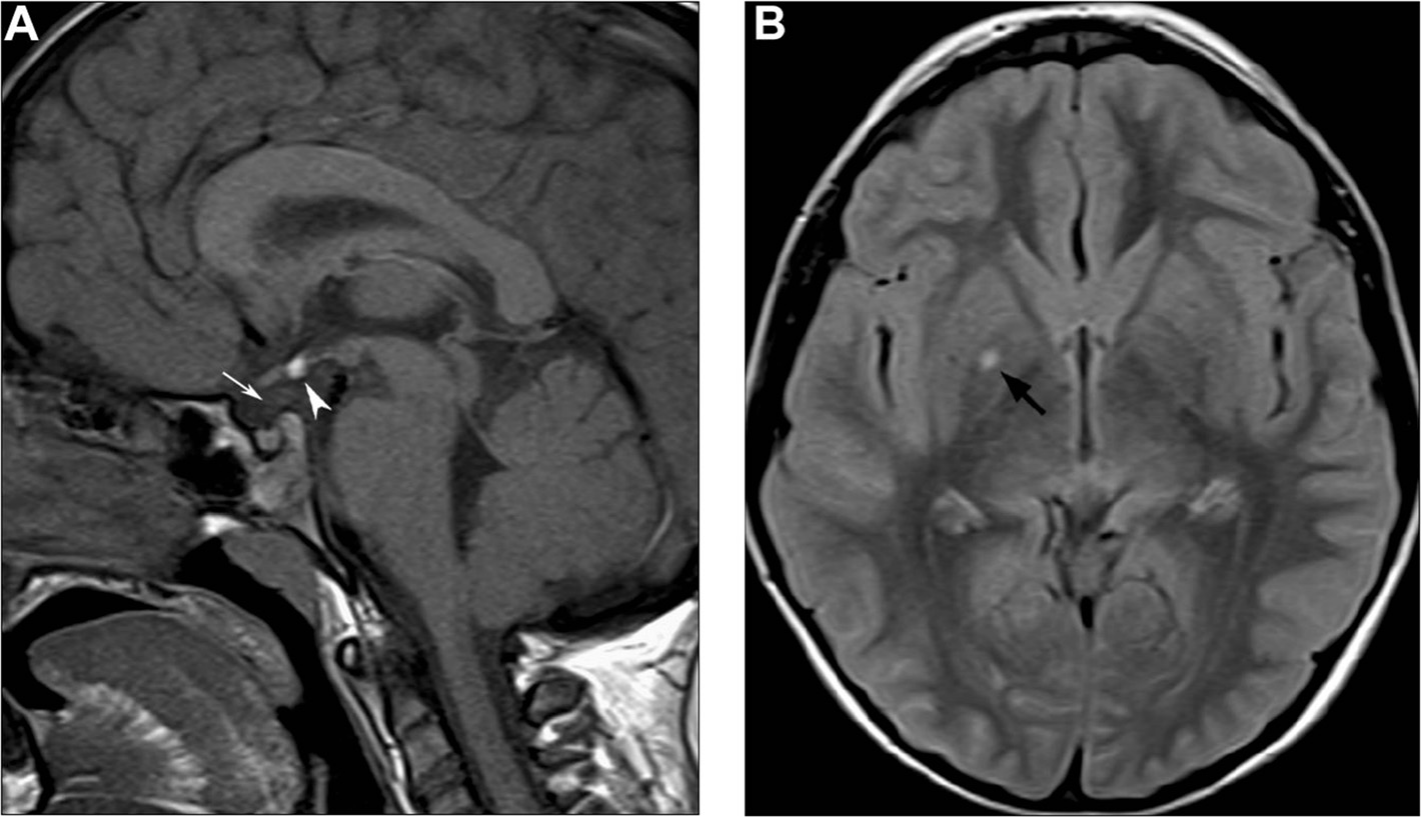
Brain MRI of the patient at 4.8 years. A) Unenhanced midline sagittal T1-weighted image shows hyperintensity corresponding to ectopic posterior pituitary lobe at the median eminence (arrowhead). The pituitary gland and sella turcica are small, and the pituitary stalk is not visible (white arrow). B) Axial FLAIR image reveals a small nonspecific hyperintense lesion in the right globus pallidus (black arrow).
